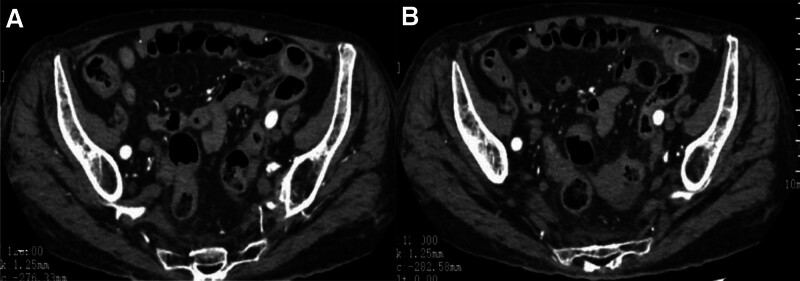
After treatment, reexamination of abdominal CT shows no obvious abnormalities (A and B). CT = computed tomography.
